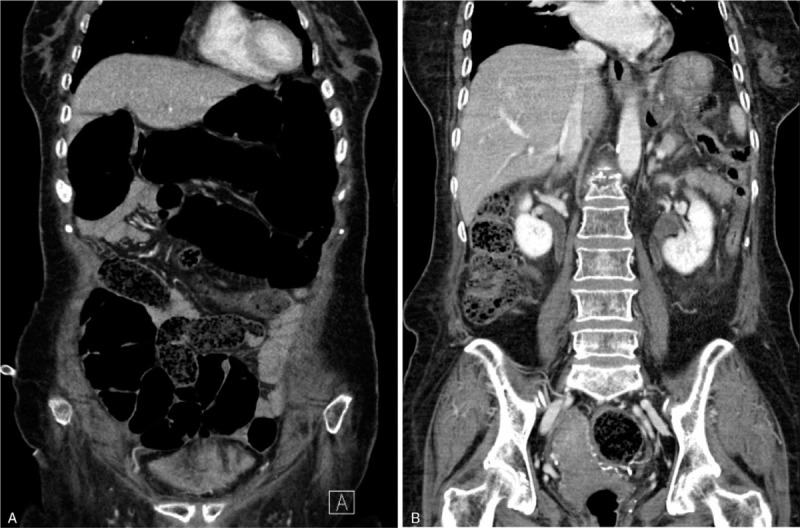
Abdominal computed tomography demonstrate paralytic ileus (A) and contrast enhancement along the renal pelvis and ureteral walls of both kidneys (B). Compression fracture of the first lumbar vertebra is also noted (B).
